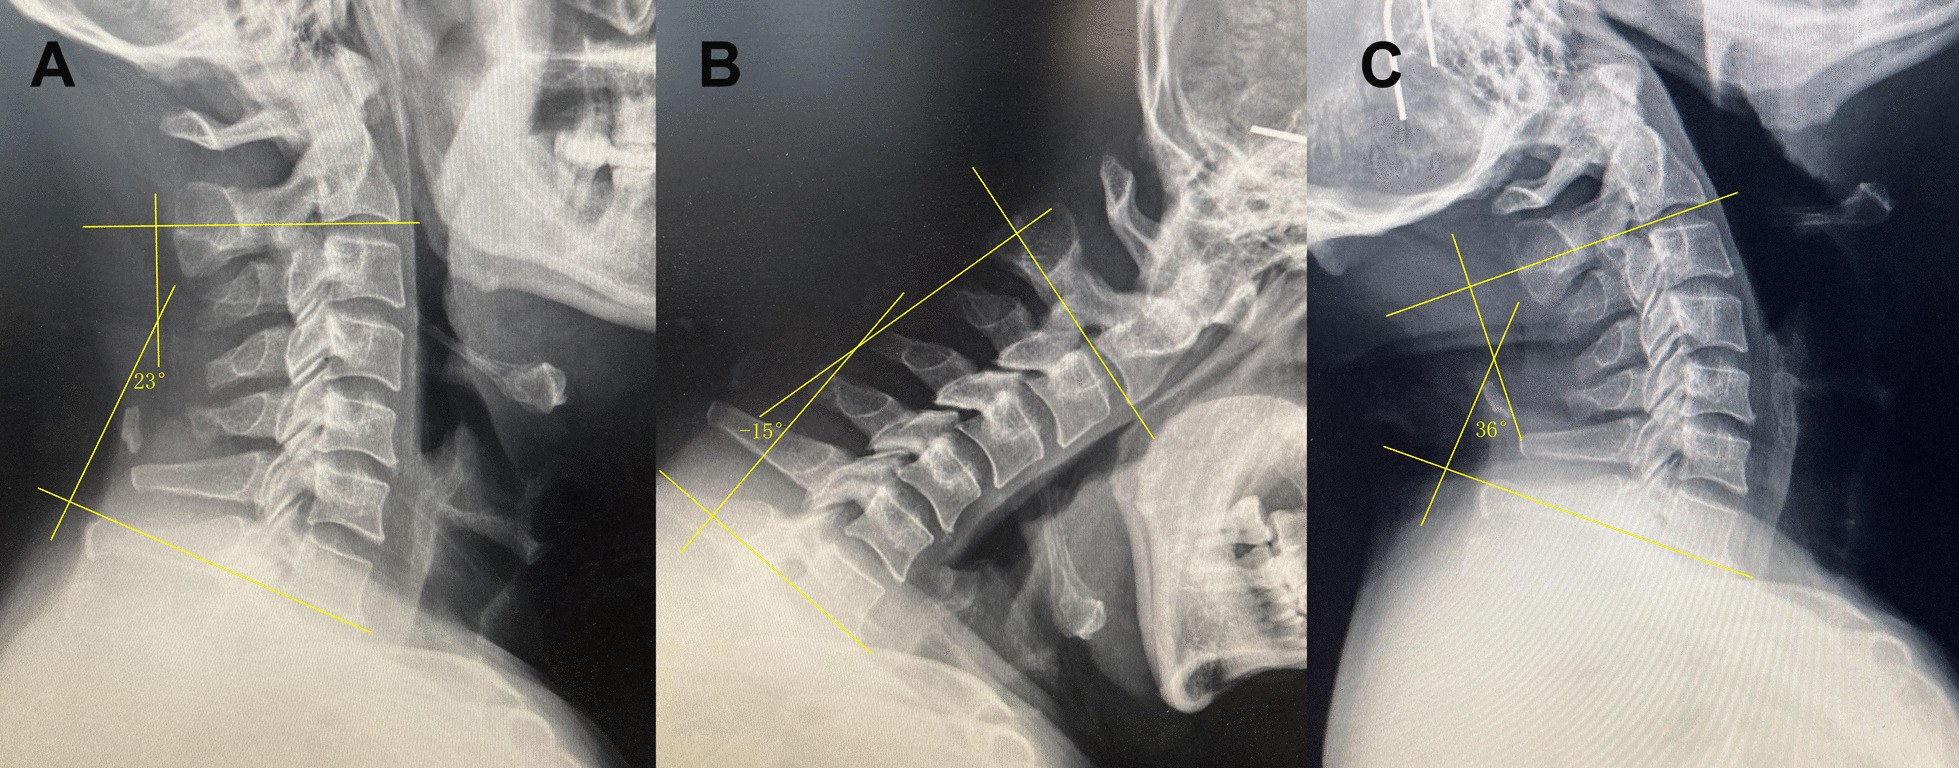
Radiologic parameters on plain radiographs of neutral (A), flexion (B) and extension (C) included C2-7 Cobb angle (CA)
